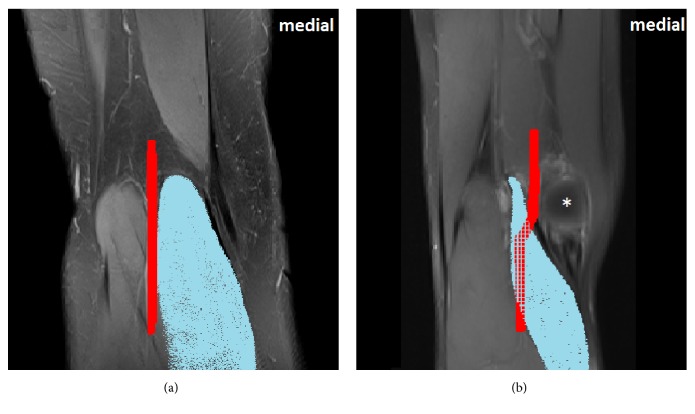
MRI of the right knee with regular anatomy (a, left) in comparison to our patient with PAES Type II (b, right). In the right image, the MHGM (light blue) is lateralized from its regular origin at the medial condyle of the femur (asterisk) and compresses the medially displaced PA (red).
